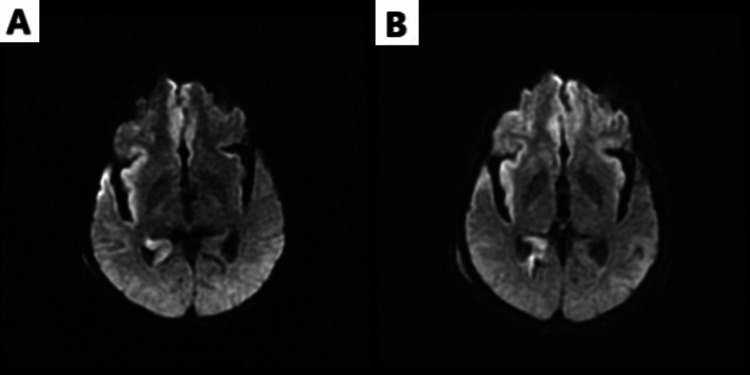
(A) MRI day 5: DWI showing mild diffusion restriction and cortical edema along the mesial frontal mid convexities, right insular cortex, right anterior temporal lobe, and right hippocampus without vasogenic edema or hemorrhage; (B) MRI day 8: DWI showing worsened restricted diffusion with subtle associated meningeal enhancement corresponding to the restricted diffusion most prominently within the right temporal lobe and insular cortex. MRI: magnetic resonance imaging; DWI: diffusion-weighted imaging.
